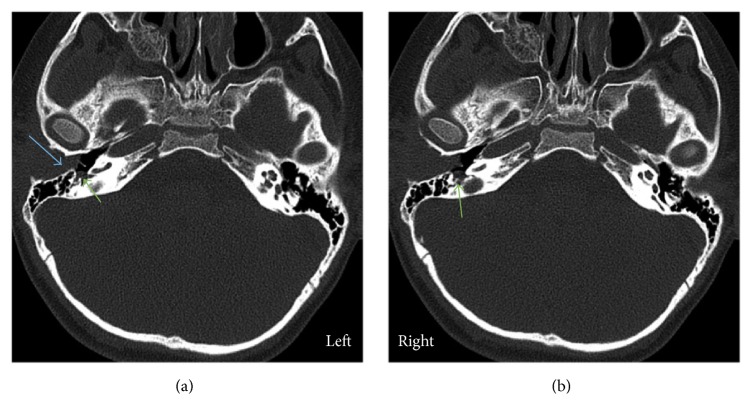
Axial CT scan. Showing soft tissue mass in the right external auditory canal with widening and occlusion of right ear canal (blue arrow) and small middle ear soft tissue opacity (green arrow).
